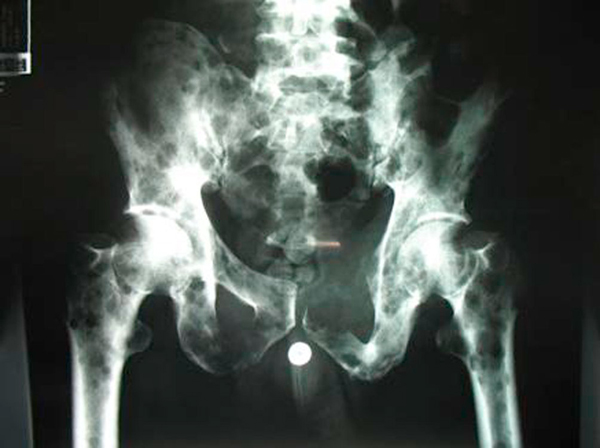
Plain radiograph of the pelvis and proximal femora showing extensive lytic lesions.
